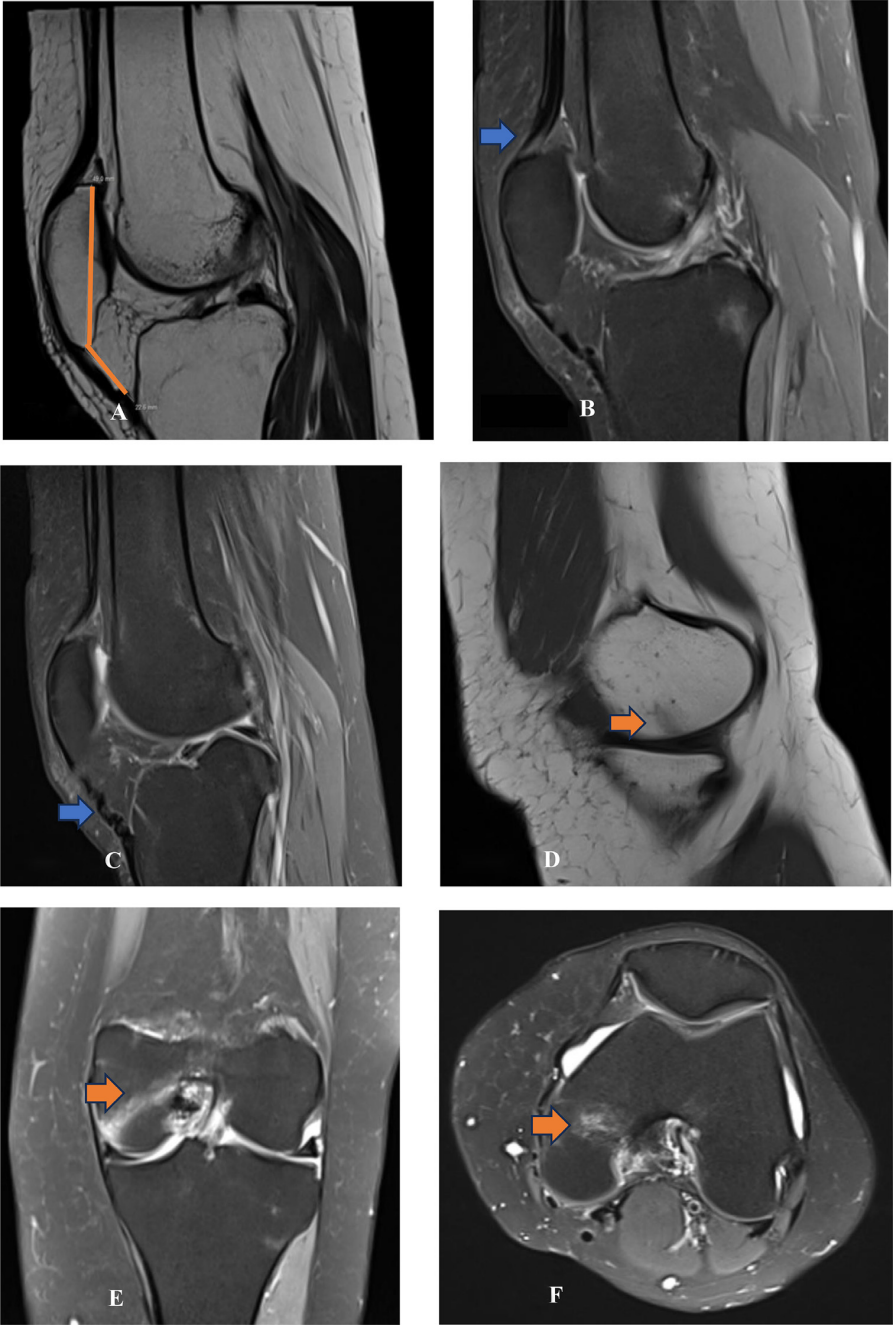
Multiplanar MRI views of the right knee (A) Sagittal T1-weighted image: Shows low patellar position with an Insall-Salvati ratio of 0.5. The patellar tendon appears thickened and tortuous. (B) Sagittal DP-weighted image: Demonstrates mild quadriceps tendinopathy, with signal alteration at the tendon insertion (blue arrow). (C) Sagittal T1-weighted image: Demonstrates a tortuous appearance of the patellar tendon (blue arrow), consistent with chronic tendinopathy. (D) Sagittal T1-weighted image: Reveals a linear subchondral fissure in the medial femoral condyle (orange arrow), suggesting focal bone injury. (E–F) Coronal DP-weighted (E) and axial T1-weighted (F) images: Reveal bone marrow edema in the medial femoral condyle (orange arrows), consistent with subchondral stress response on multiple planes.
